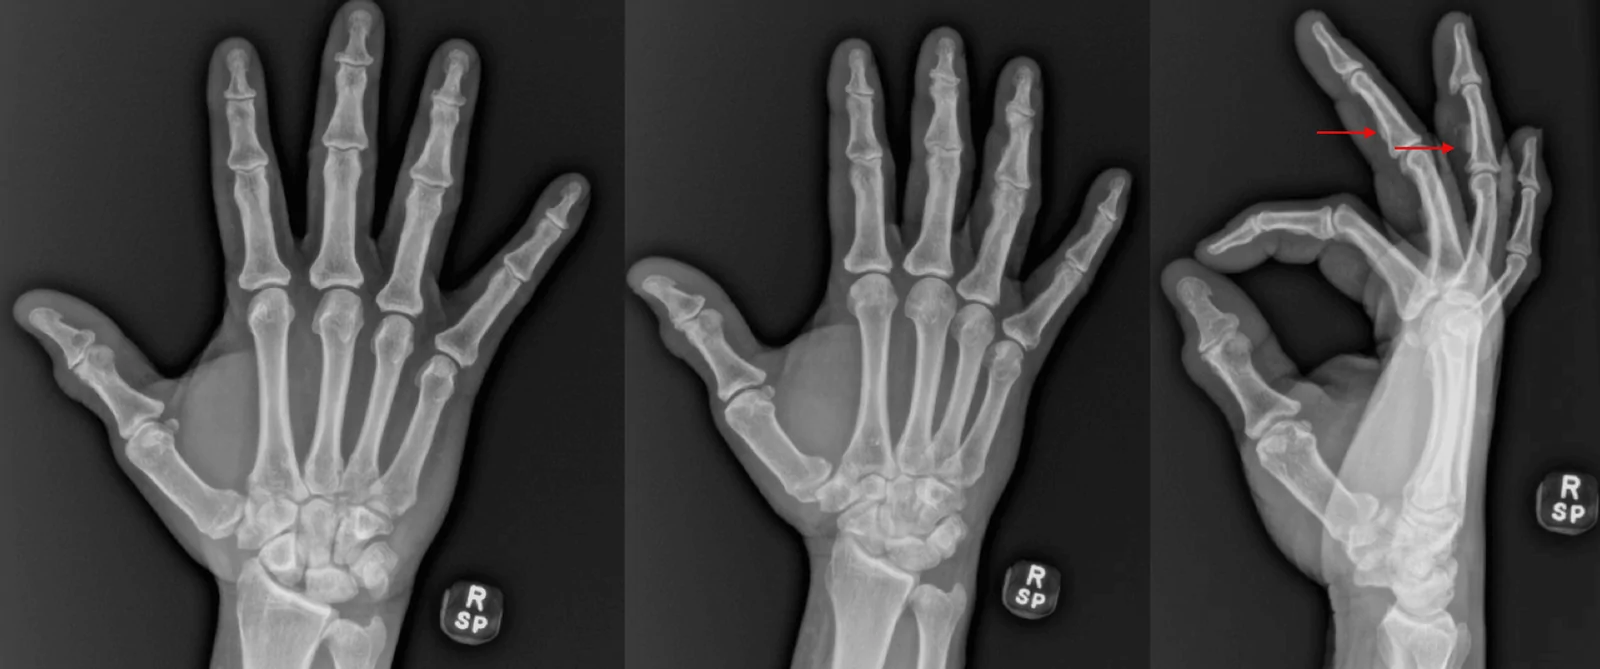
Anteroposterior, oblique, and lateral radiographs of the right hand demonstrate palmar osseous spurs at the base of the middle phalanges of the long and ring fingers (arrows on the lateral view), as well as arthritic changes in the distal interphalangeal joint of the ring finger, including joint space narrowing and a palmar osteophyte. A smaller osseous spur is also present at the base of the middle phalanx of the index finger.
